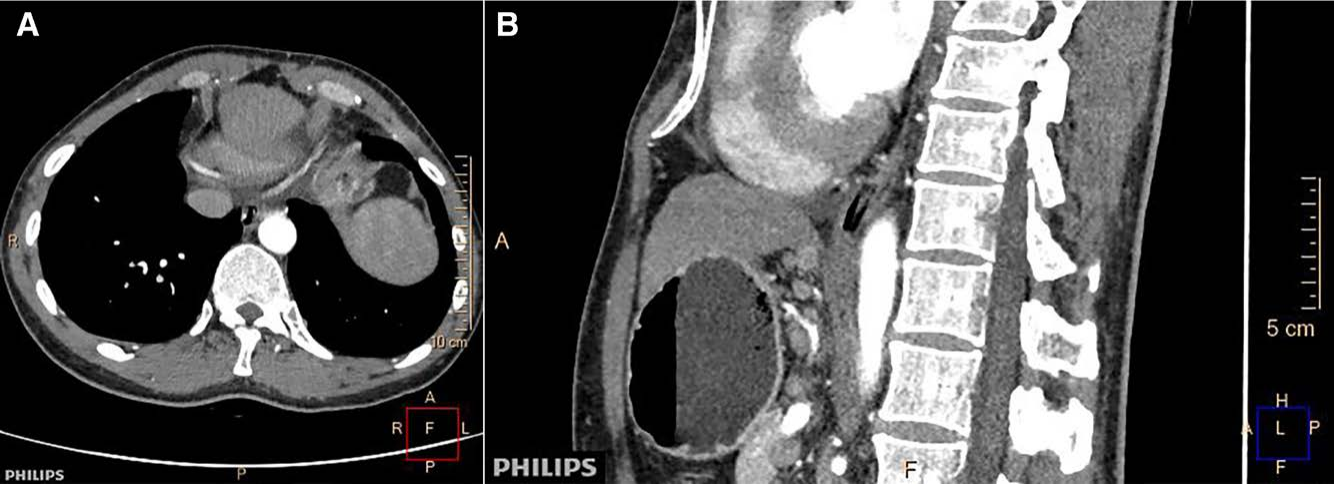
Enhanced computed tomography of the chest confirmed the dissection with air within the esophageal wall.
